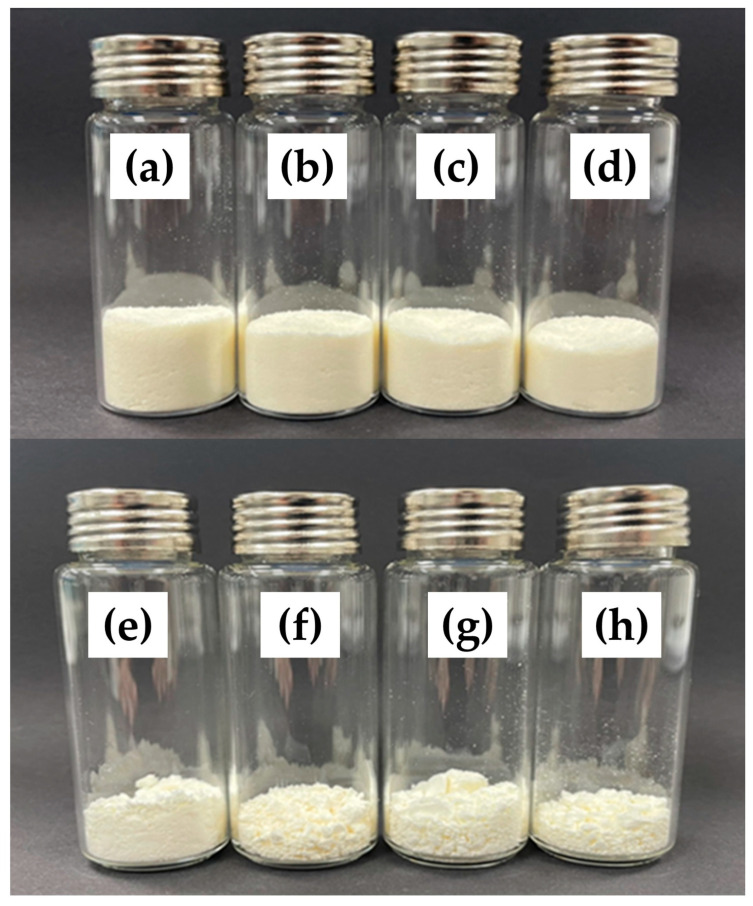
Appearance of the prepared Yuja dried gels: SDG-Y-NE/MD (a), SDG-Y-NE/MD/βCD (b), SDG-Y-NLC/MD (c), SDG-Y-NLC/MD/βCD (d), FDG-Y-NE/MD (e), FDG-Y-NE/MD/βCD (f), FDG-Y-NLC/MD (g), and FDG-Y-NLC/MD/βCD (h).
